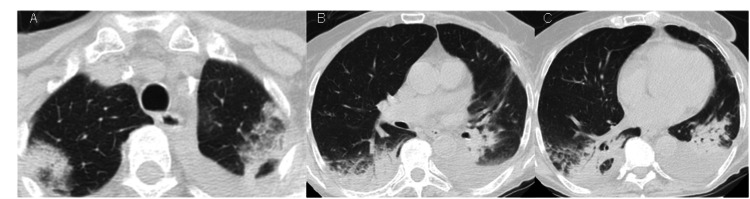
Chest CT on day 14 after admission. Persisting bilateral pulmonary consolidations with aerial bronchogram despite antibiotic therapy escalation.
